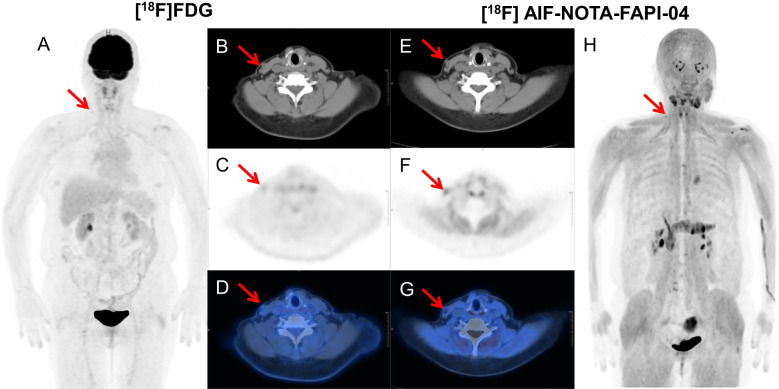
A 60-year-old woman with follicular thyroid cancer who had undergone total thyroidectomy 8 years ago. Her thyroglobulin level was elevated to 85.92 ng/mL. [^18^F]FDG PET/CT demonstrated a node with SAD of 6mm in right cervical level III with mild FDG uptake (SUVmax: 2.0, arrow). [^18^F]AlF-NOTA-FAPI-04 PET/CT showed moderate tracer accumulation in the corresponding region (SUVmax: 4.0, arrow). Subsequent lymph node biopsy revealed metastatic thyroid carcinoma. [^18^F]FDG PET/CT images (A–D) and [^18^F] AlF-NOTA-FAPI-04 PET/CT images (E–H): maximum-intensity projections (A, H), axial CT (B, E), PET (C, F), and fused PET/CT (D, G) are shown. SAD, short-axis diameter; SUVmax, maximum standard uptake value.
